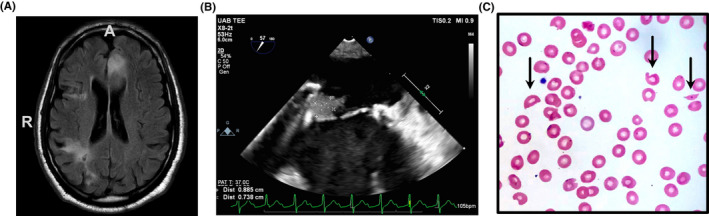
(A) Axial T2 FLAIR magnetic resonance imaging demonstrating the acute left ACA distribution infarction and multiple prior infarctions. (B) Two‐dimensional trans‐esophageal echocardiography of prosthetic mitral valve demonstrating a 1.5 cm vegetation. (C) Peripheral blood smear prior to initiation of plasma exchange therapy
